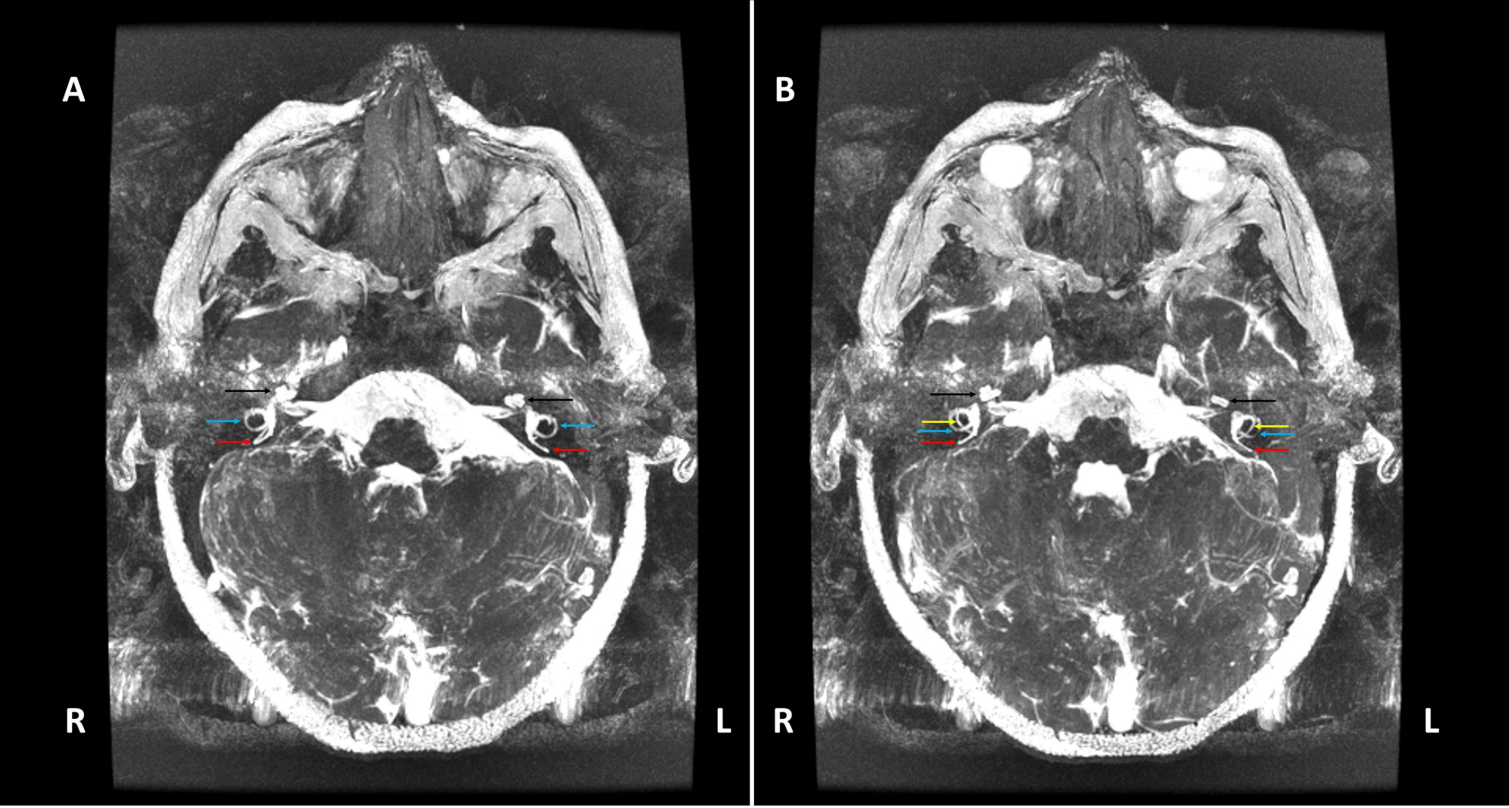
Axial MIP reconstruction of the FIESTA MRI images of the IAC. A) The image demonstrates preserved fluid signal, bilaterally, within the cochlear turns (black arrows), as well as within the posterior (red arrows) and lateral (blue arrows) semicircular canals. B) The subsequent image also demonstrates preserved fluid signal, bilaterally, outlining the posterior (red arrows), lateral (blue arrows), and anterior (yellow arrows) semicircular canals, as well as within the cochlear turns (black arrows). On the left (L) side, the cochlea appears smaller compared with the cochlea of the right (R) side. MIP: maximum intensity projection; FIESTA: fast imaging employing steady-state acquisition; MRI: magnetic resonance imaging; IAC: internal auditory canal; R: right side; L: left side.
